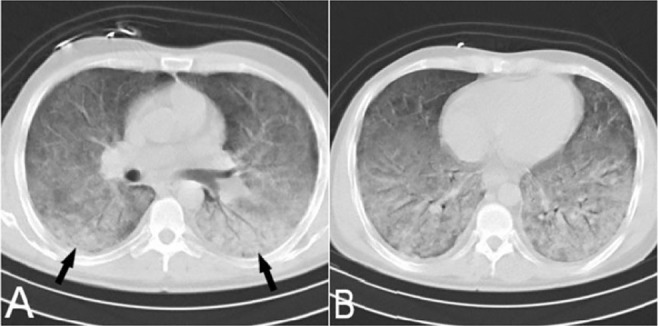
A,B)Non-contrast axial chest CT scan of 36-year-old man, a case of HIV who developed dry cough and fever showed bilateral GGO more dominant in para-hilar locations with no sign of effusion and no lymphadenopathies. PCR result for Bronchoalveolar lavage fluid (BALF) was positive for Pneumocystis pneumonia.
